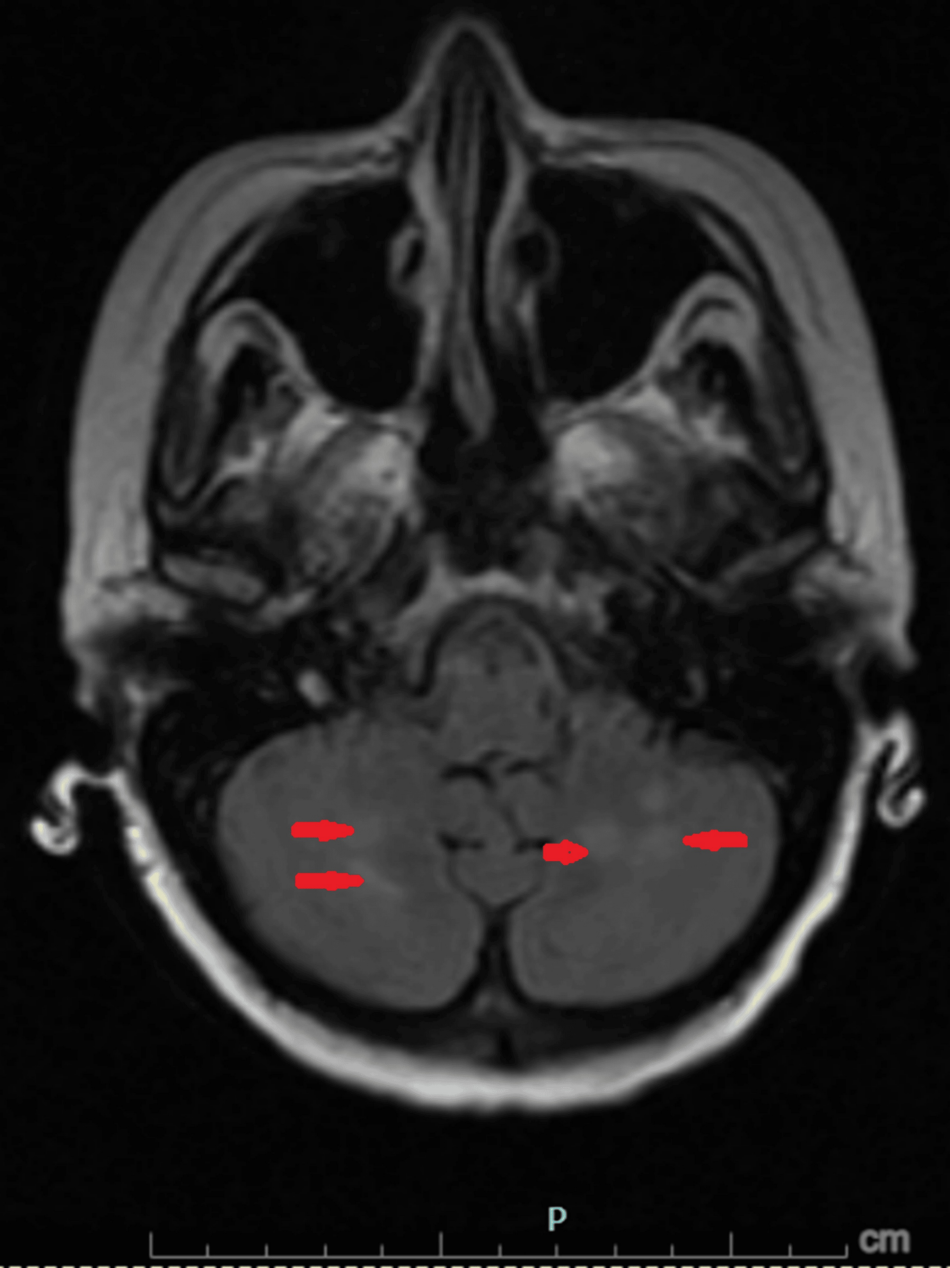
Cerebellar involvement in posterior reversible encephalopathy syndrome Fluid-attenuated inversion recovery (FLAIR) MRI images of the brain showing symmetric involvement of the bilateral cerebellar hemispheres (red arrows), consistent with posterior reversible encephalopathy syndrome in the appropriate clinical context.
